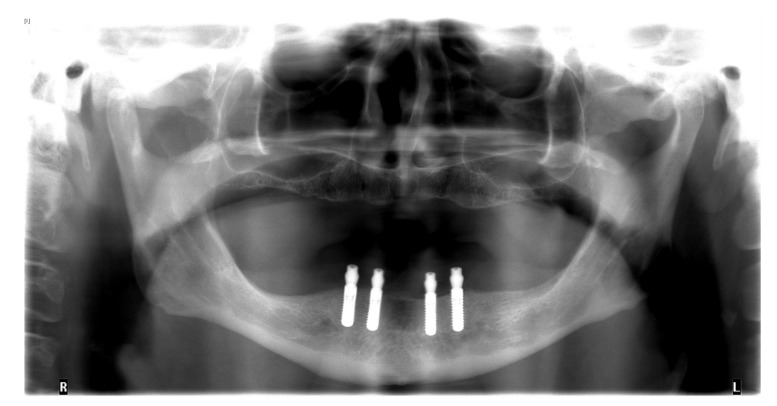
Orthopantomogram after insertion of four implants in the lower jaw.
